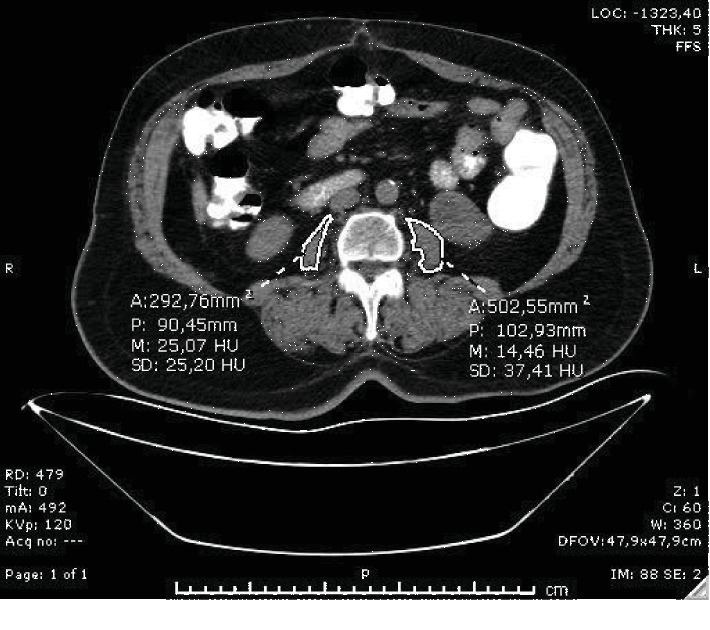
65-year-old woman with pulmonary embolism. The value of psoas muscle area was obtained by dividing the sum of the right and left psoas muscle areas at the level of L3 vertebrae into body surface areas using single axial CT scan images. He had higher PESI scores, lower arterial oxygen saturation, and higher respiratory rate in-hospital mortality.
